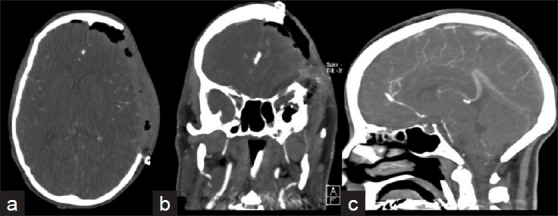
(a) Axial, (b) coronal, and (c) sagittal angio-CT performed immediately after surgery; an aneurysm pointing antero-superiorly and originating from the distal ACA (A3 segment) at its bifurcation with the callosomarginal artery was visualized
